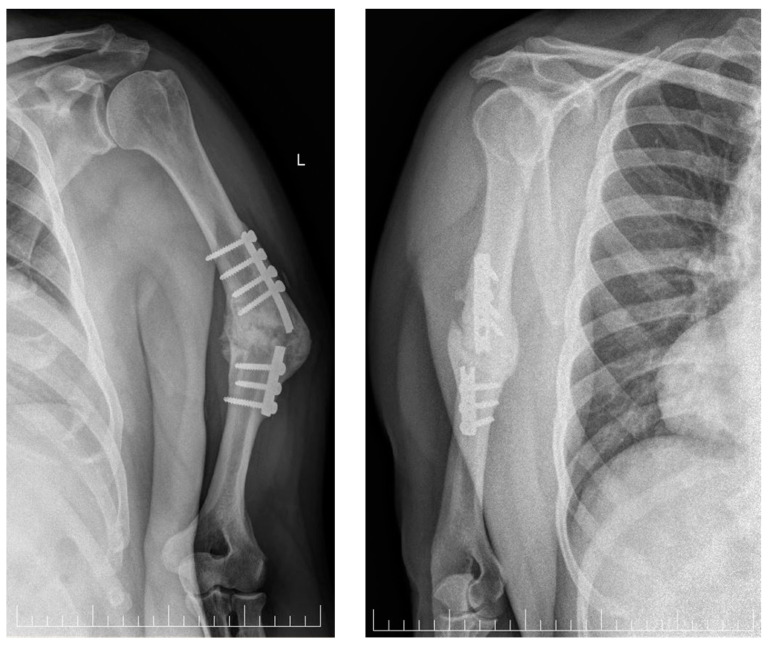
Pre-operative X-ray of the left humerus. Left: antero-posterior view, and right: latero-lateral view, showing hypertrophic nonunion of the humeral shaft fracture with the presence of deteriorated osteosynthesis material.
